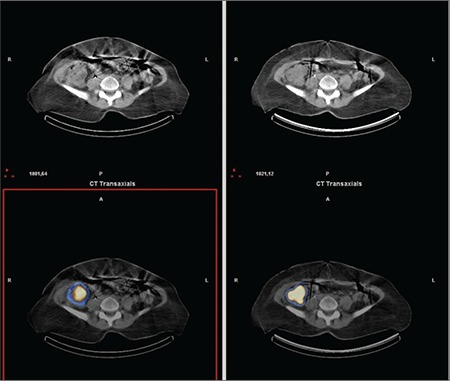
Comparison of early and late phase SPECT/CT images; transaxial slices
